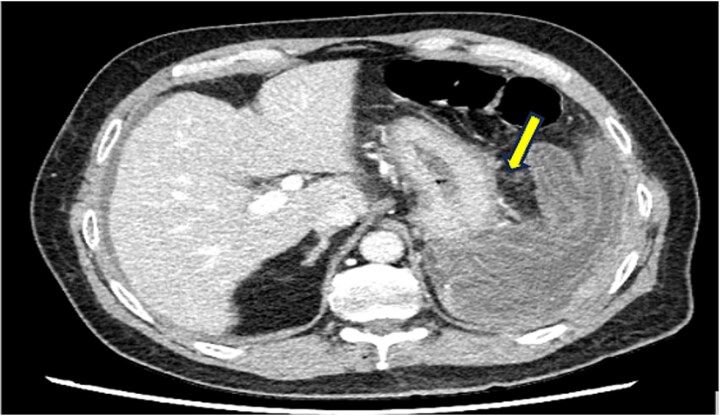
Axial contrast-enhanced CT image demonstrating inflammatory fat stranding surrounding the splenic lesion, with associated free intraperitoneal fluid.
